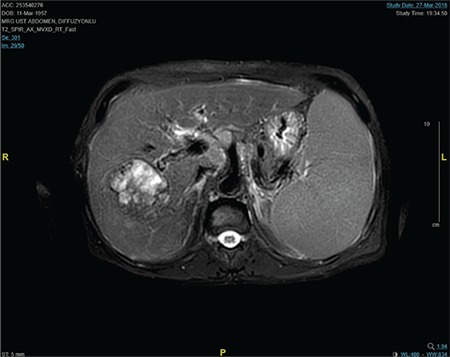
Hepatic tumor in abdominal magnetic resonance imaging.
